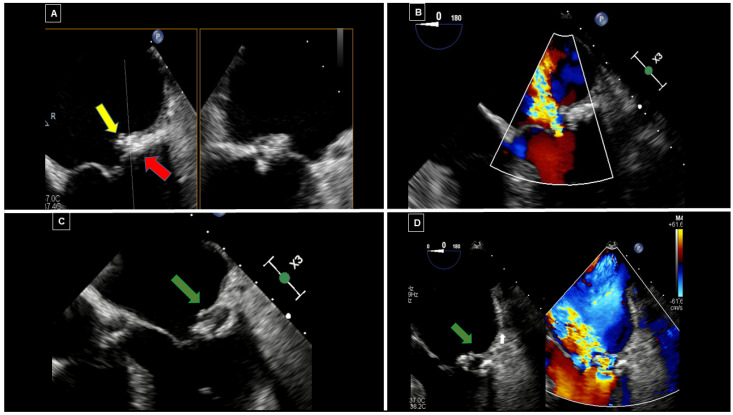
Evolution of MAC towards CCMA with fistulzation at TOE. Transesophageal echocardiography (TOE) black and white (A,C) and color-Doppler mode (B,D) images at mid-esophageal 0° view, acquired at first evaluation (A,B) and after 2 weeks (C,D) in a 77-year-old woman with infective endocarditis of the mitral valve due to Methicillin-susceptible Staphylococcus Aureus (MSSA) with cerebral embolization.
